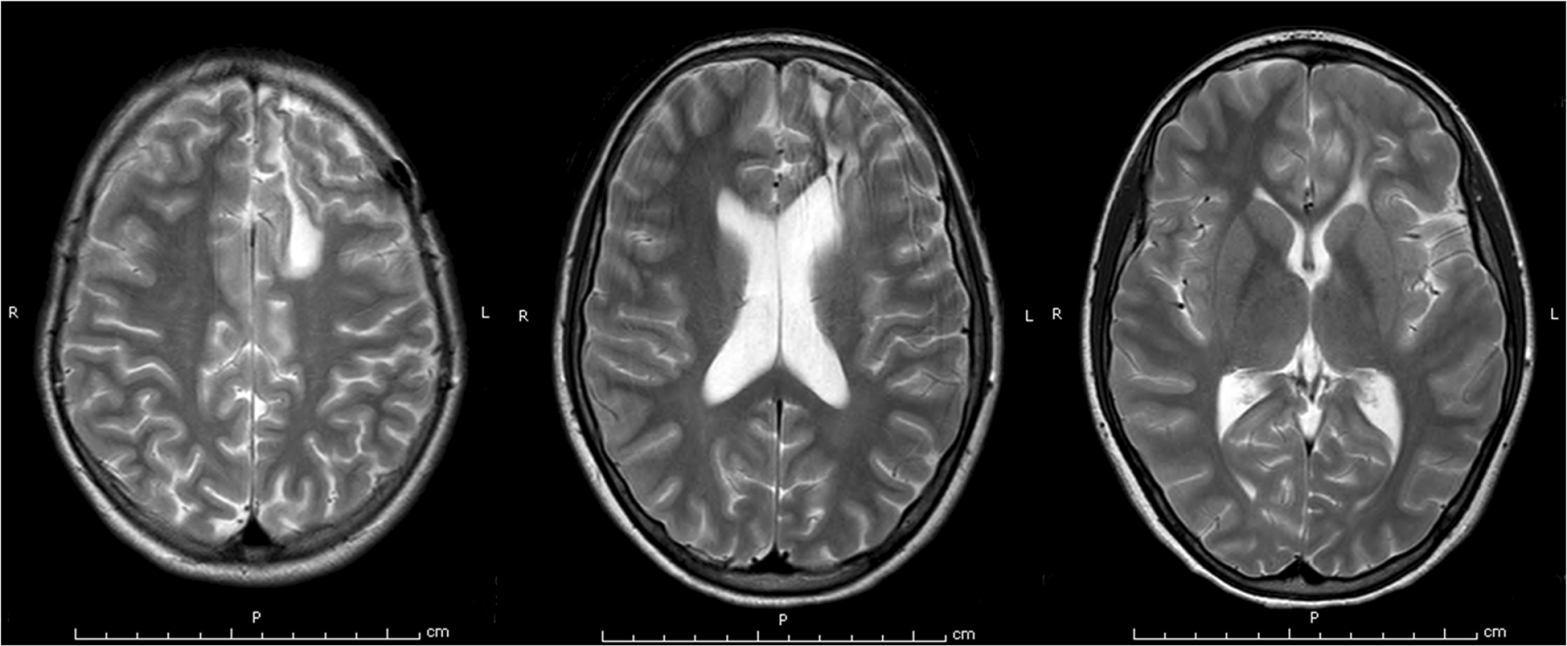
Brain MRI of case 2. T2-weighted images on brain MRI showing, in the left supratentorial region, the known surgical outcomes (with left frontal craniotomy) and a malacic area, communicating with the omolateral frontal horn. No further significant signal alterations are evident.
